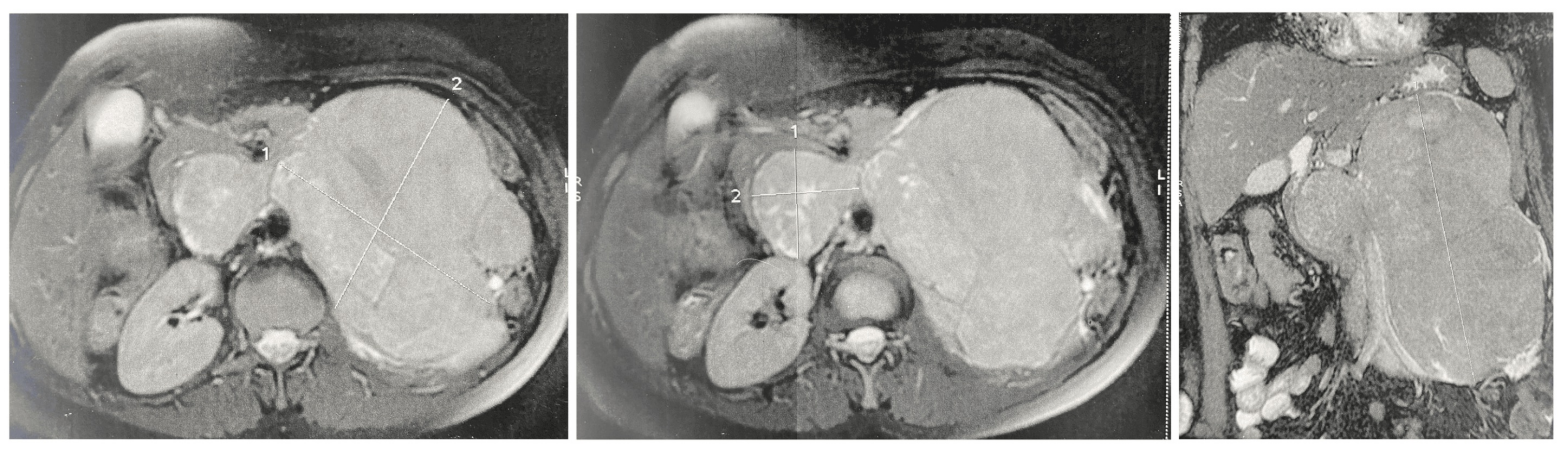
Abdominal-pelvic MRI images showing the dimensions of the mass (215 x 122 x 177 mm) and its intimate relationship with the aorta, left kidney and left urethra
